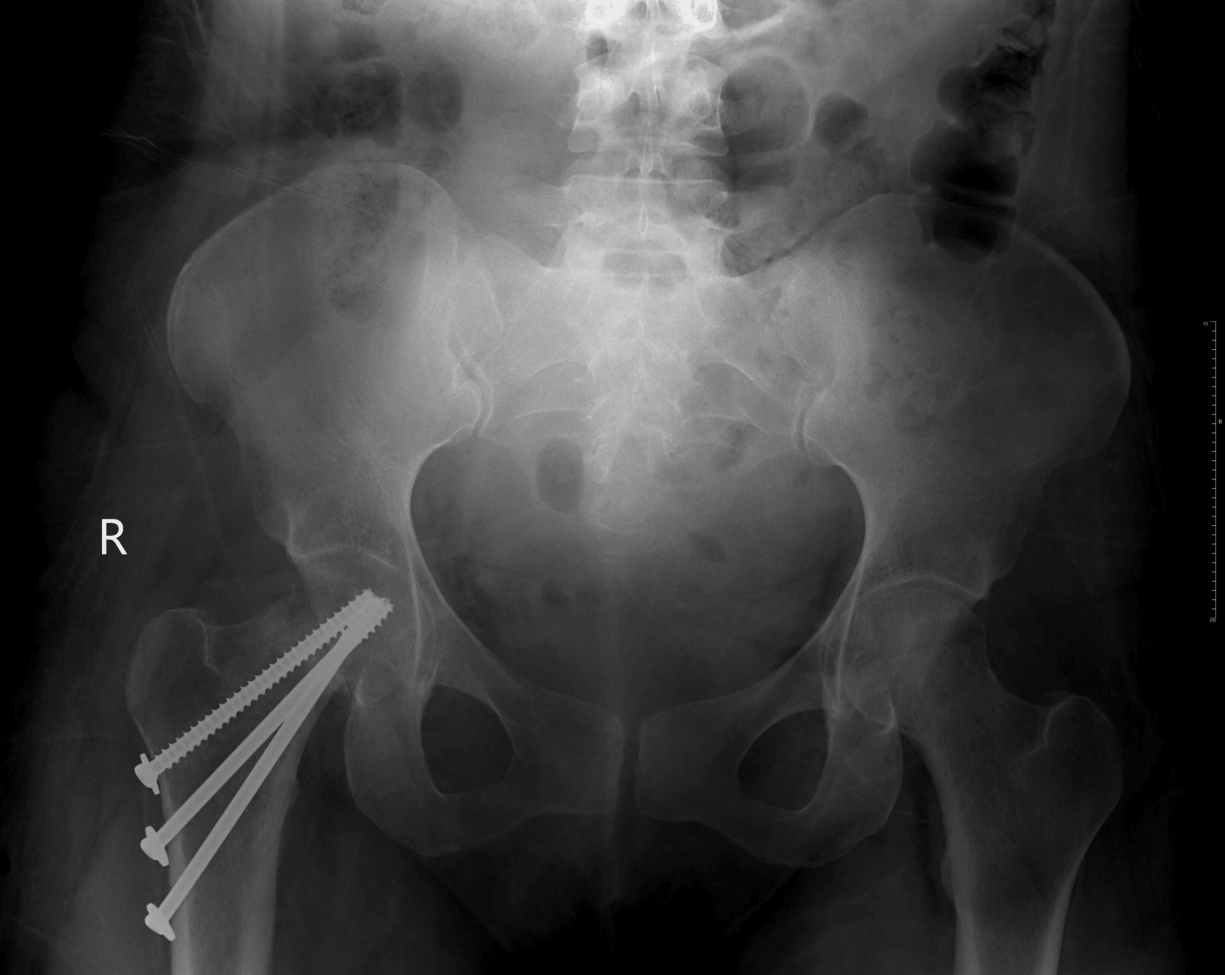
Post-screw fixation check X-ray The check X-ray shows acceptable fixation after percutaneous screw fixation of the right femoral neck.
